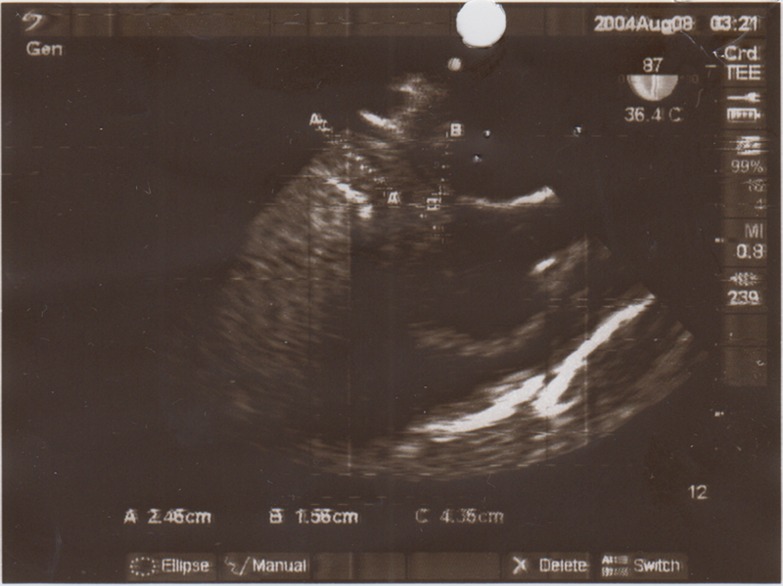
Echocardiography view showed thrombosis in the entrance to the inferior vena cava to the right atrium.
